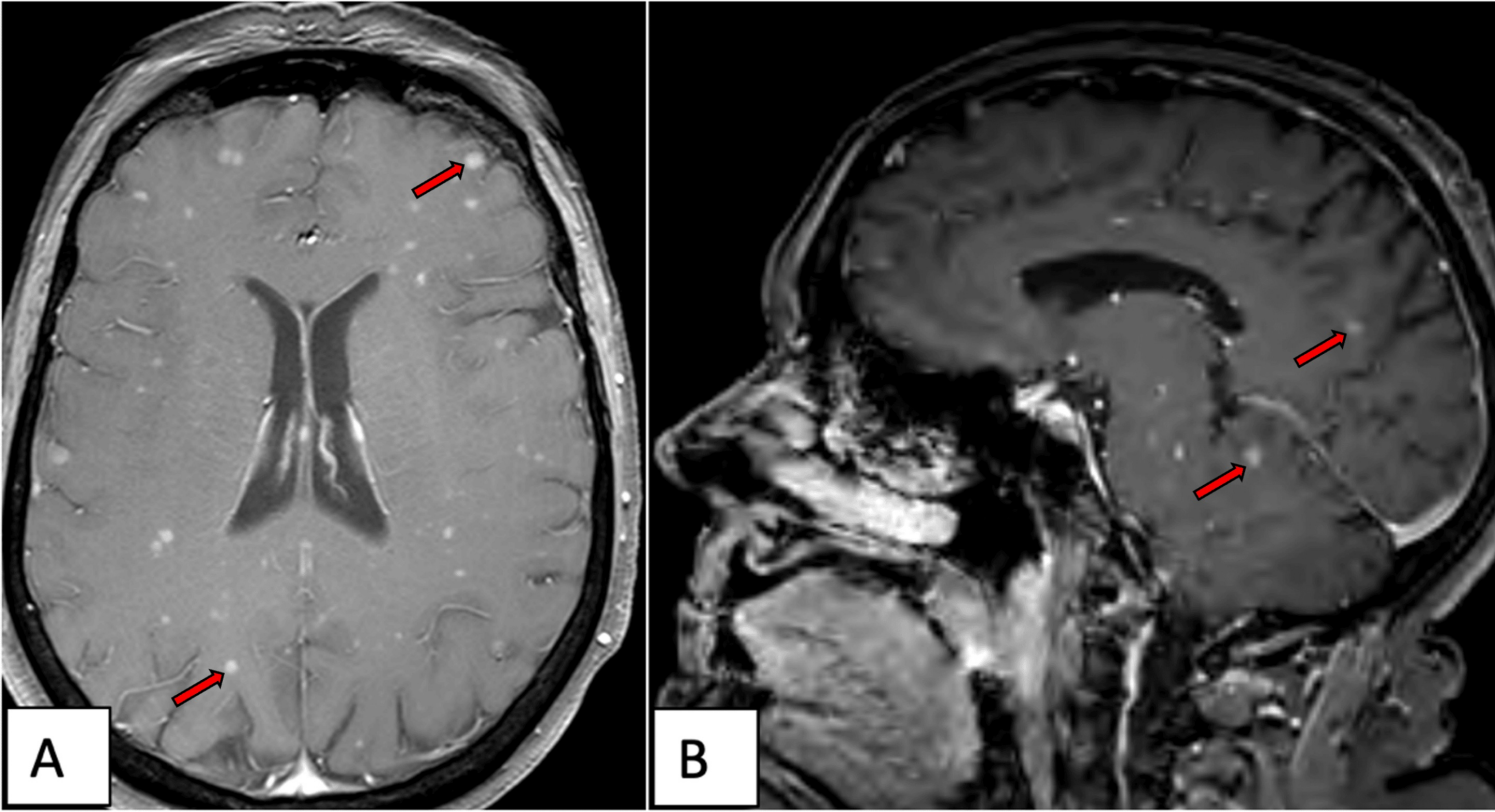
Brain MRI detailing widespread supratentorial and infratentorial atypical carcinoid metastases (red arrows) that progressed through systemic therapy. A) Axial and B) sagittal views
